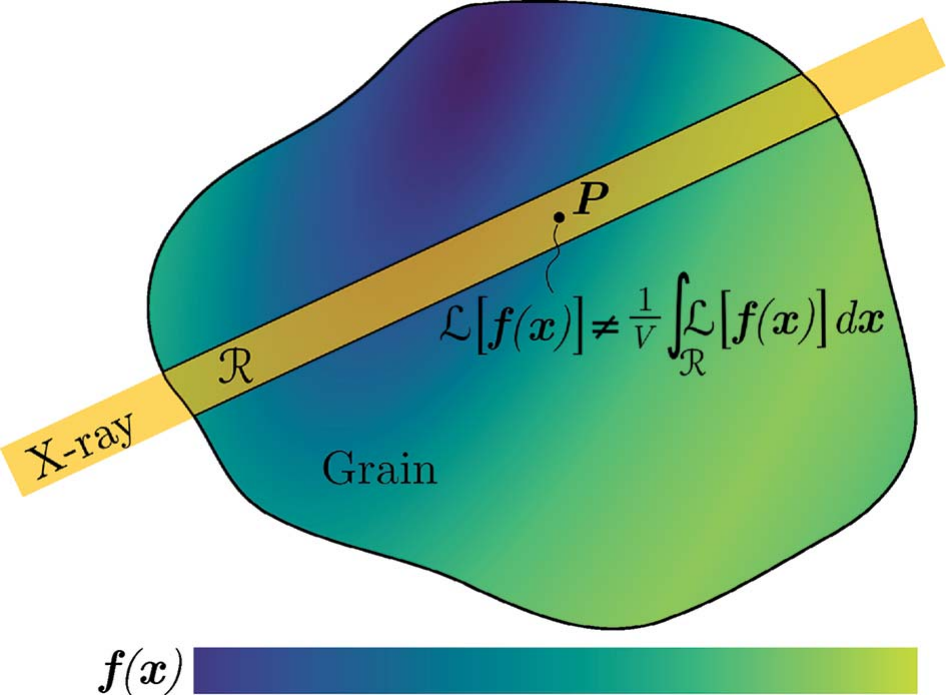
X-ray measurement from a region
